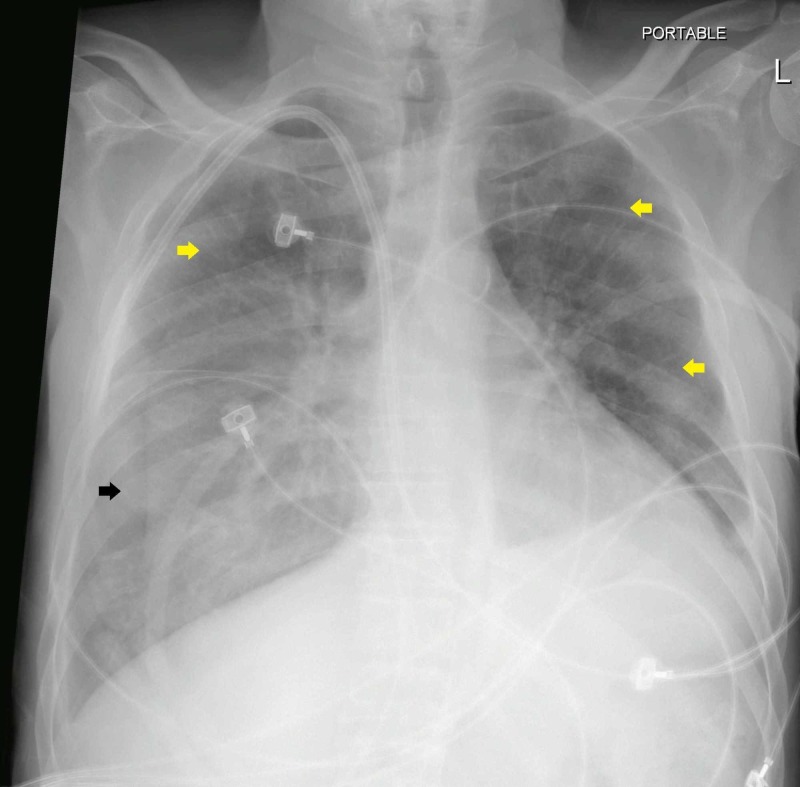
Portable chest x-ray (14 days after admission) showing partial interval resolution of the opacities in the left lung and upper zone of the right lung (Yellow Arrows). Remaining opacities are visible in the right lower lobe (Black Arrow).
